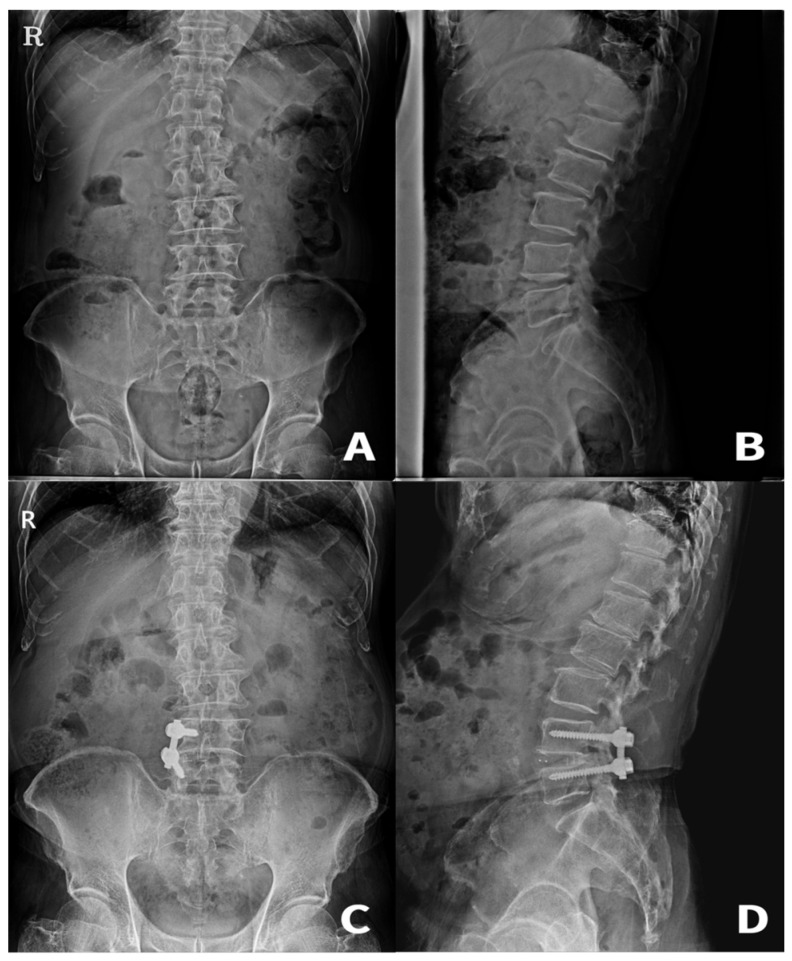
A 72-year-old male patient who underwent unilateral instrumented transforaminal lumbar interbody fusion for spinal stenosis combined with disc extrusion causing right-sided radiating pain unresponsive to conservative treatment. (A,B) Preoperative radiographs. (C,D) Radiographs at 65 months after surgery showing maintenance of the lumbar alignment.
